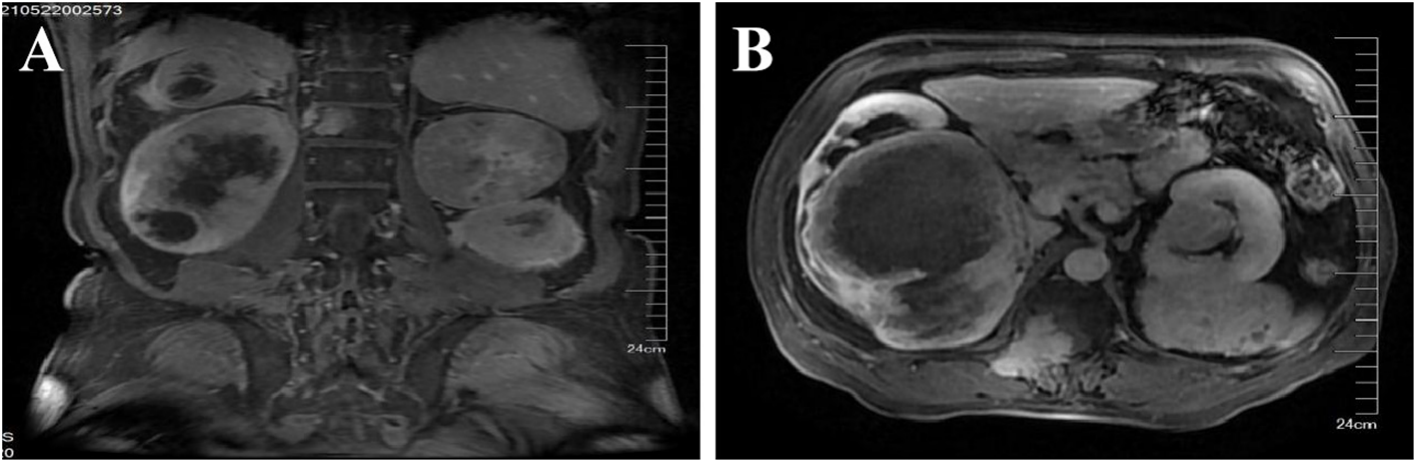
MRI of both kidneys. Both kidneys are enlarged in volume and irregular in shape, and multiple round and irregular masses can be seen, with uneven enhancement. The larger one is located in the right kidney, with a size of about 179 × 121 × 135 mm, and the bilateral renal pelvis is deformed by compression. Bone metastasis of chest 12, right adnexal area, and right sacrum may occur. (A) Sagittal position. (B) Coronal position.
